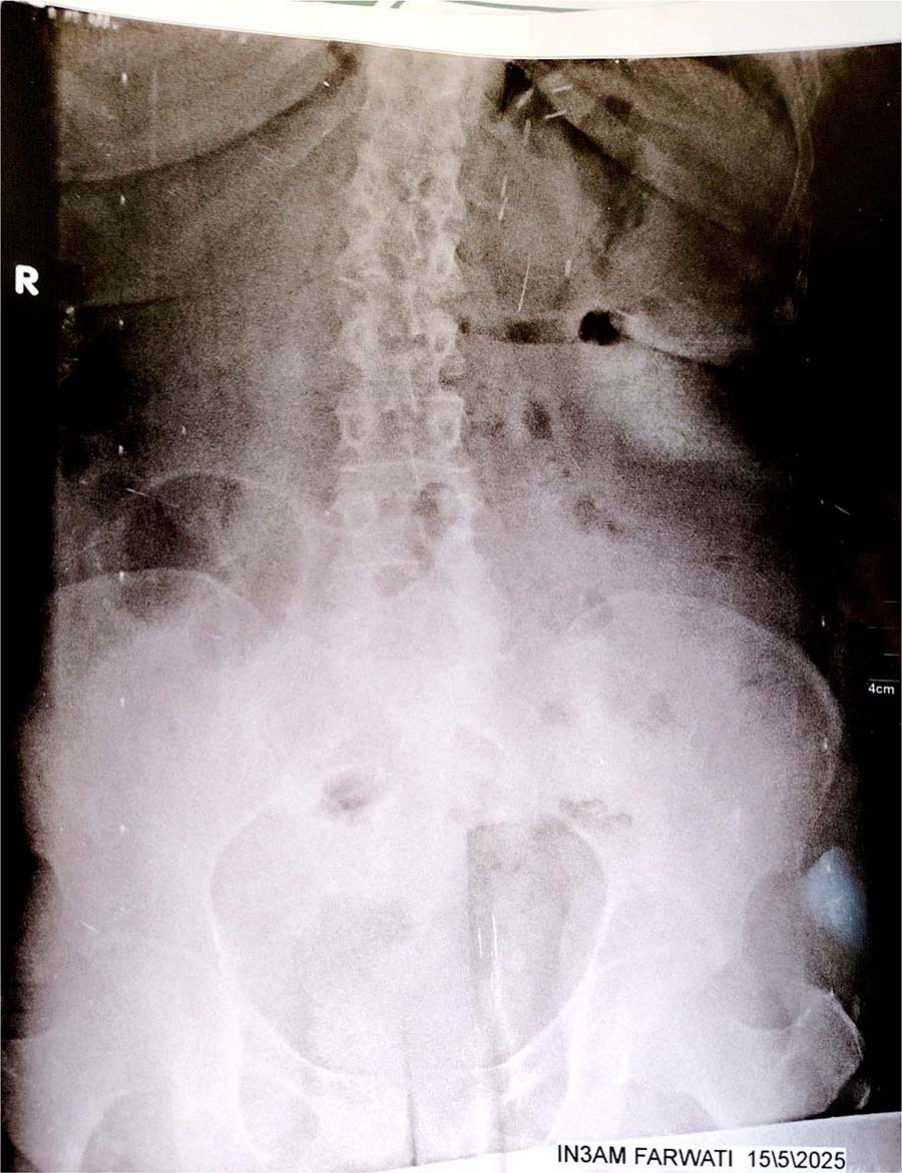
Plain abdominal X-ray (upright position) showing prominent colonic and small bowel gas patterns with no visible air-fluid levels or signs of acute obstruction. No abnormal bowel positioning or dilation is clearly evident. These findings were non-specific and contributed to the diagnostic delay.
